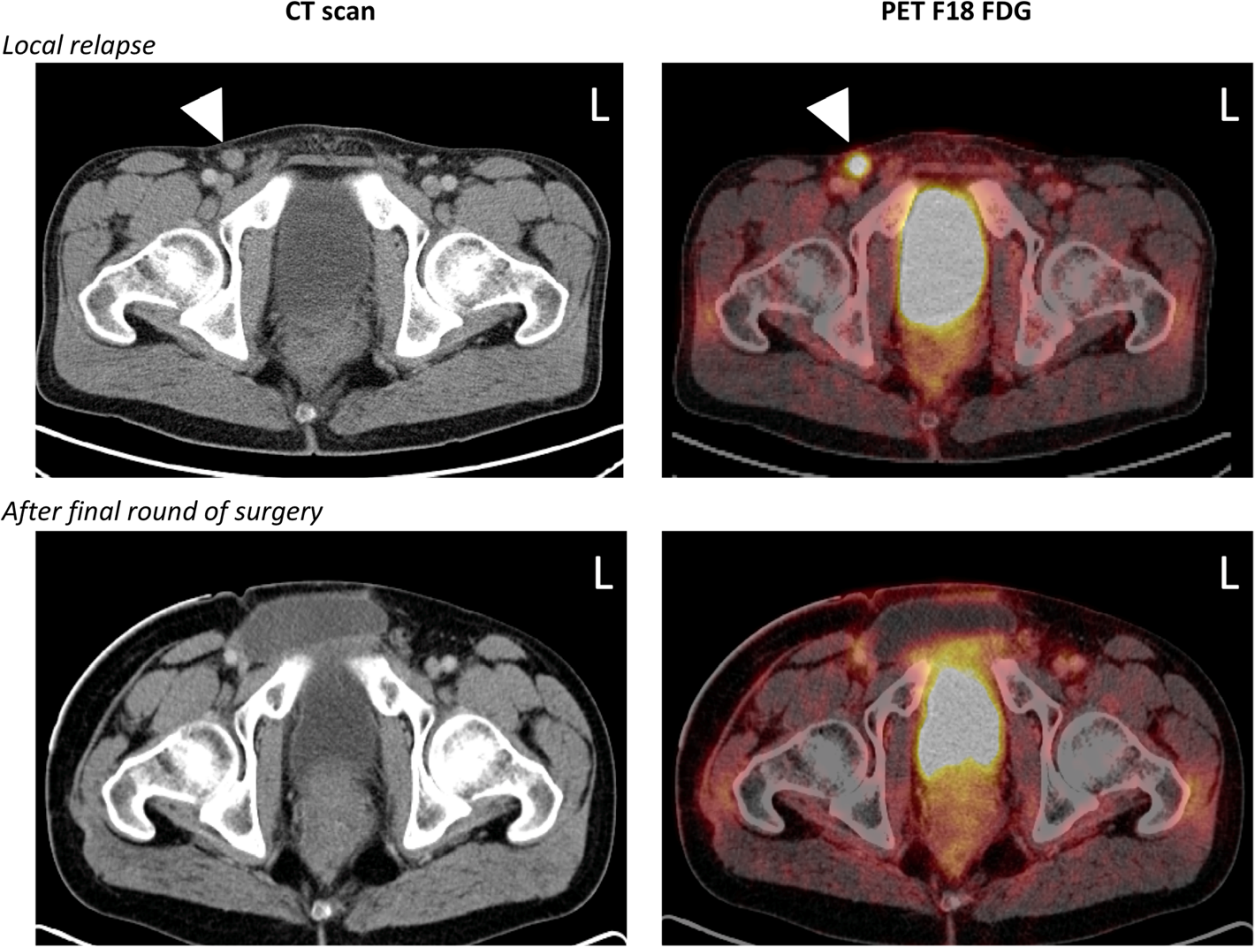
Imaging of the lower abdominal cavity and pelvic region visualizing one of the local recurrences as well as the radiological evidence of complete remission after the final round of surgery. Left columns depict conventional computerized tomography (CT) scans and right columns visualize the positron emission tomography with fluorodeoxyglucose (PET F18 FDG) findings. Top row: White arrowhead highlights one of the local recurrences, a right-sided subcutaneous inguinal lymph node metastasis. Bottom row: No remaining tumor tissue is evident after the fourth and final round of surgery. Left side is marked by the letter “L”
